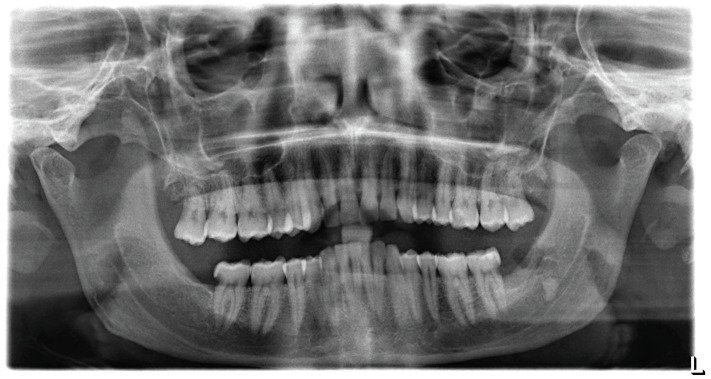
Panoramic radiograph taken after the lower wisdom teeth extraction, shown on the right side the residual fractured root.
